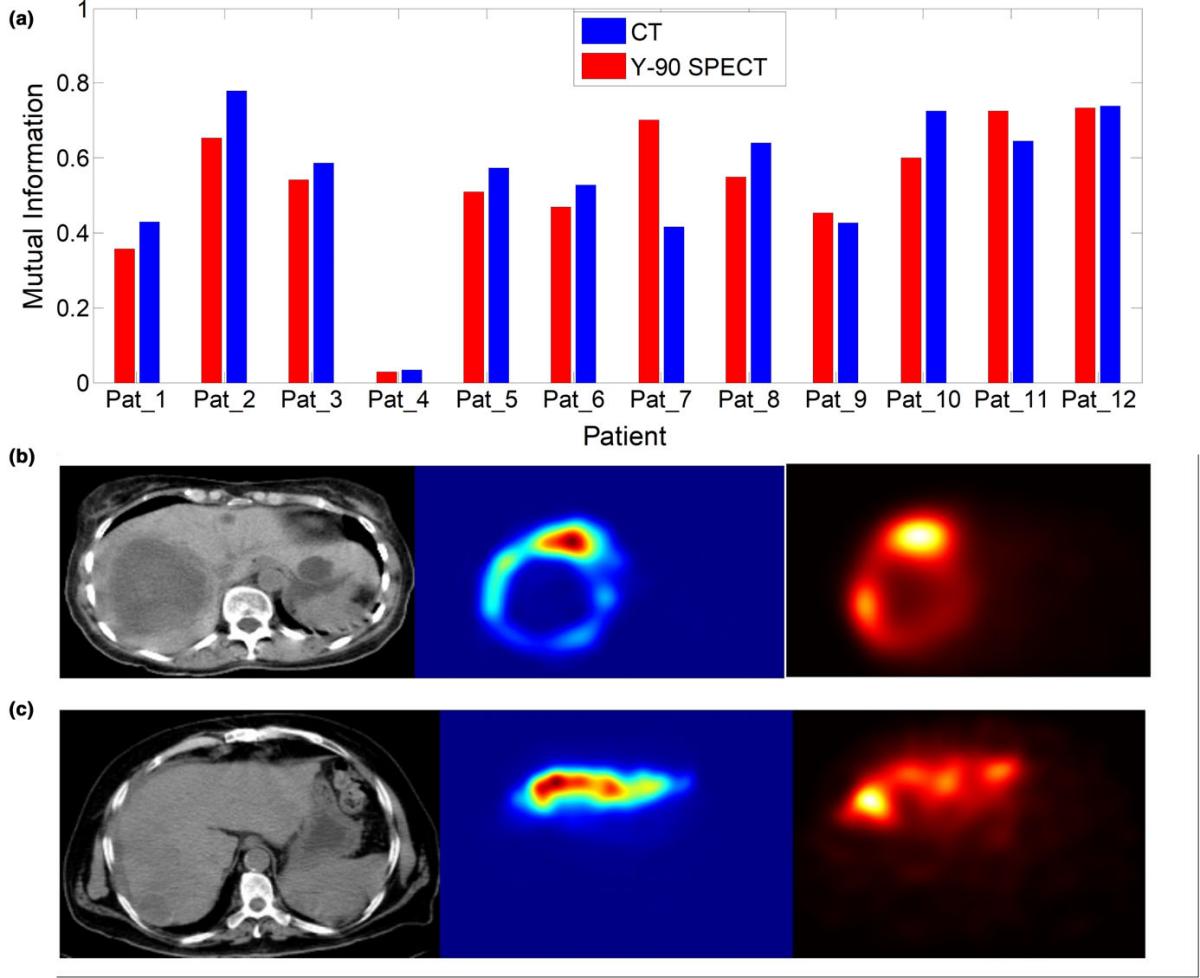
(a) Mutual information between co‐registered 90Y microsphere and 99mTc‐MAA SPECT images. (b) Patient 7 computed tomography (CT) (left), 99mTc‐MAA (middle) and 90Y microsphere (right) SPECT scans showing a minimal uptake within the liver. (c) Patient 4 CT (left), 99mTc‐MAA (middle) and 90Y microsphere (right) SPECT that showed the smallest mutual information between the co‐registered pre and post‐treatment images.
